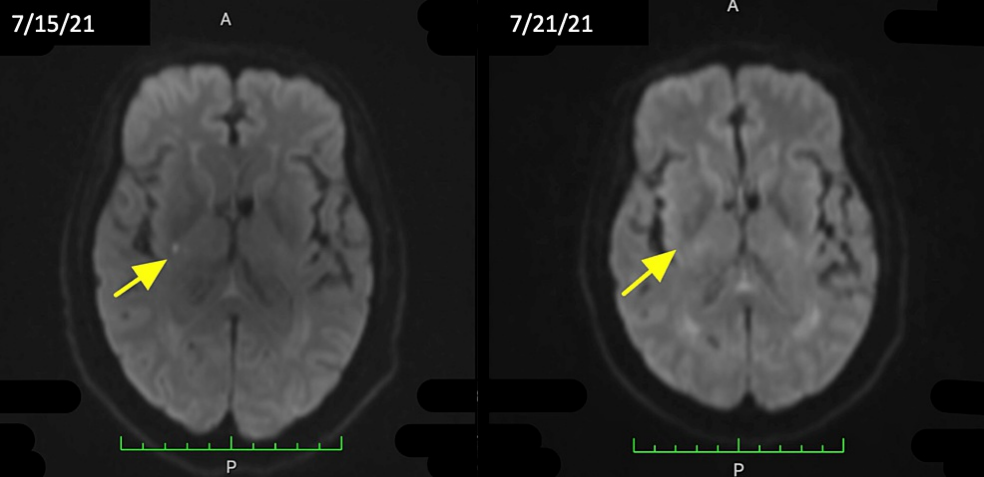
Patient's brain MRI showing punctate focus of restricted diffusion in the right posterior limb of the internal capsule consistent with an acute lacunar infarct (left). Infarct no longer seen (right).
